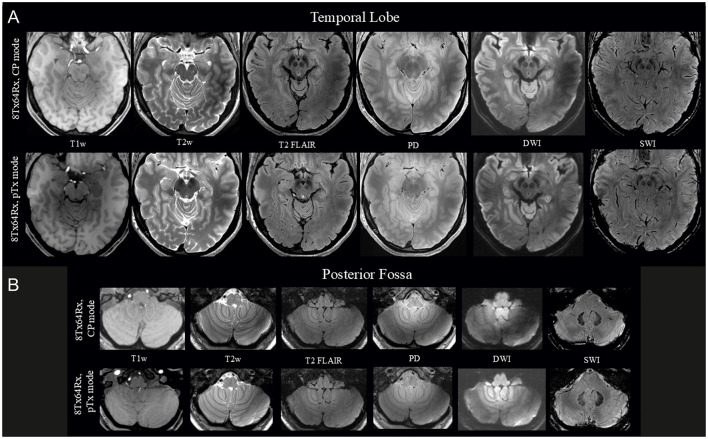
Comparison of images acquired in a different healthy volunteer (compared to Figure 12) with various sequences (axial 3D T_1_-weighted, T_2_ TSE, T_2_ FLAIR, PD, DWI, and SWI) at the level of temporal lobes (A) and cerebellum/posterior fossa (A), obtained with the 8Tx64Rx (CP) (top rows) and 8Tx64Rx (pTx) (bottom rows), respectively.
